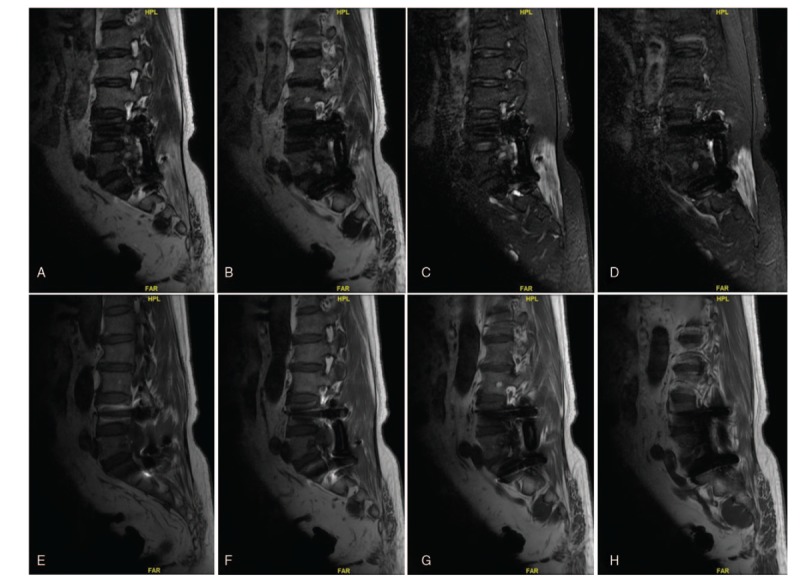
(A–H) Sagittal plane of the magnetic resonance imaging scan revealed the tumor in the spine, with high suspicion of the recurrent pathogenic tumor.
